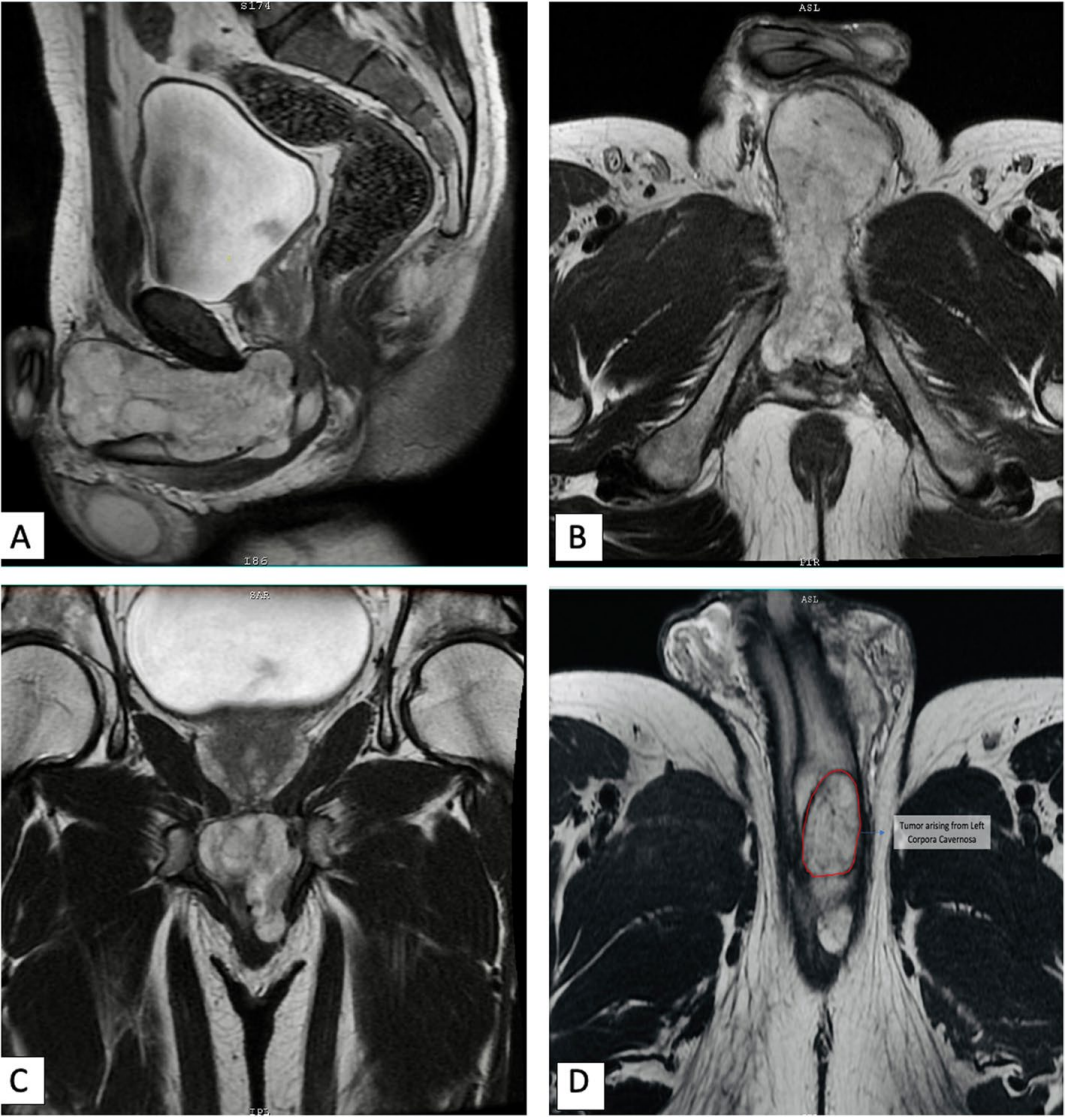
Contrast enhanced Pelvis MRI images (A)-Saggital, (B) & (D)- Axial and (C)-Coronal cuts
